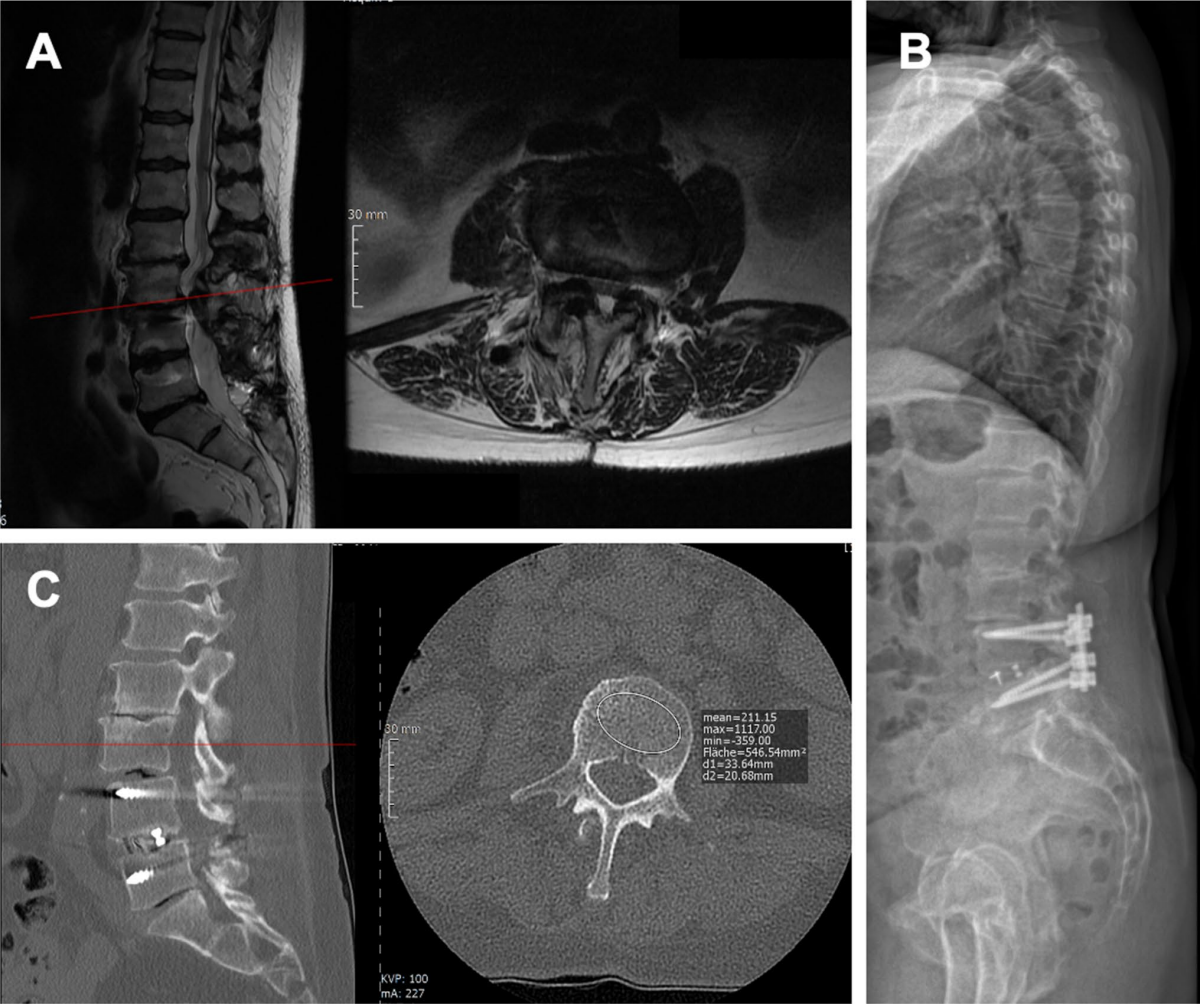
Radiological imaging of a typical patient. A MRI imaging. Left, sagittal. Right, axial; B long standing X-ray; C CT-scan. Left, sagittal. Right, axial
